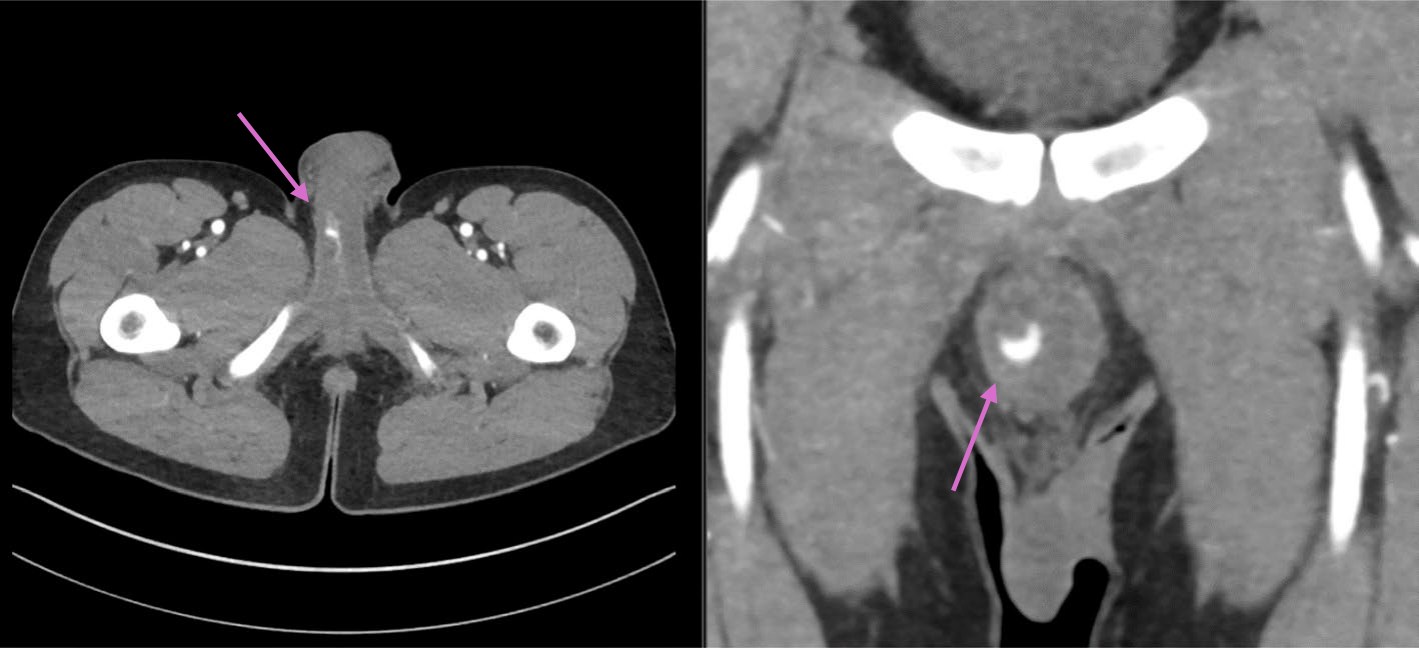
A contrast-enhanced computed tomography (CT) scan of the pelvis displaying a pseudoaneurysm located in the right cavernous body
